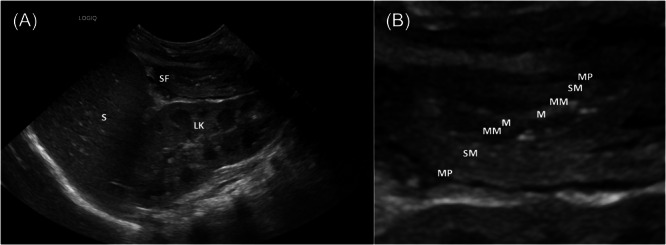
(A) Ultrasound image of the left upper quadrant on presentation imaging (Day 2) showing S, LK and SF. (B) Zoomed section from the same image showing the thickened splenic flexure of colon, indicating inflammation. The gut wall signature is outlined from innermost to outermost: echogenic M, hypoechoic MM, intermediate SM, hypoechoic MP. The serosa is not visible. The most inflamed layer at this time is the submucosa. The displayed left hemicolon was thick‐walled measuring up to 4 mm compared to the right hemicolon at 1.5 mm (not displayed). LK, left kidney; M, mucosa; MM, muscularis mucosa; MP, muscularis propria; S, spleen; SF, splenic flexure of colon; SM, submucosa.
